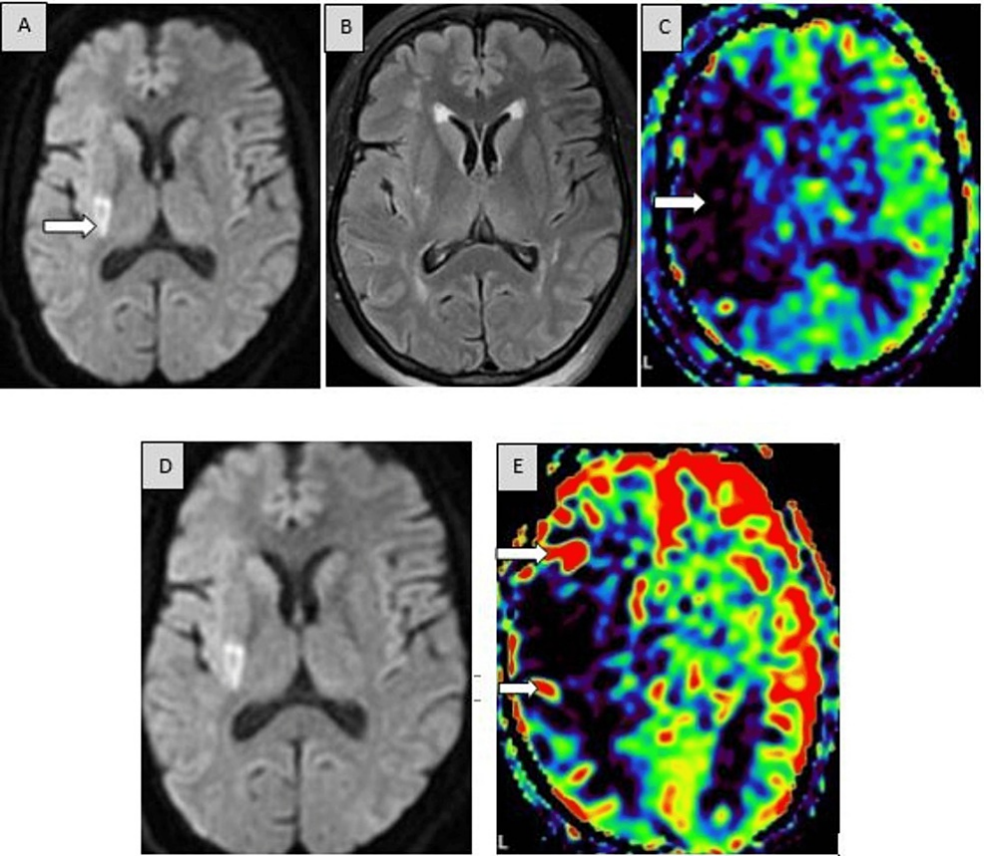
Pre- and post-thrombolysis images showing right MCA infarct with restoration of perfusion on timely thrombolysis. (A) MRI brain DWI shows a small area of diffusion restriction in the right posterior putamen (arrow). (B) Axial FLAIR image; note no significant corresponding signal changes seen in the right putamen, suggestive of a DWI-FLAIR mismatch (represents an infarct within the window period). (C) Axial 3D color coding of ASL demonstrates a large perfusion defect (arrow) in the right cerebral hemisphere in the MCA territory out of proportion to the infarct volume (A), suggestive of a DWI-ASL (perfusion) mismatch, i.e., a large peri-infarct potentially salvageable ischemic penumbra. (D) DWI in the same patient, thrombolyzed in the window period. Post-thrombolytic DWI and ASL images show the volume of infarct in the right putamen remains the same with no further progression. (E) ASL shows restoration of perfusion in the ischemic penumbra zone in the right cerebral hemisphere (arrows).
